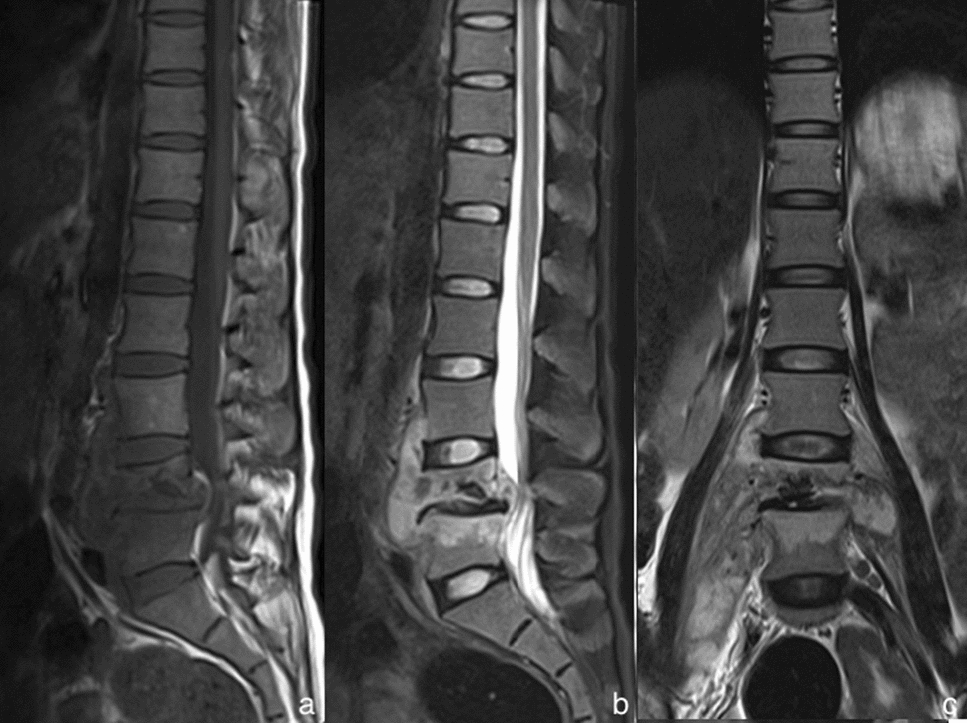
The MRI of a 23-year-old woman complaining of continuous lumbago with tuberculous spondylitis. The sagittal MRI T1WI (a), the sagittal MRI with fat saturation (b) and the coronal MRI T2WI (c) presented a combination of MRI findings: the lesions, in lumbar vertebral bodies 4 and 5, showed hypointense signal in T1WI and hyperintense signal in T2WI. They revealed typical vertebral bodies destruction and collapse, along with the vertebral posterior convex deformity and a narrowed intervertebral space. There also existed extensive psoas abscess and paraspinal abscess around
